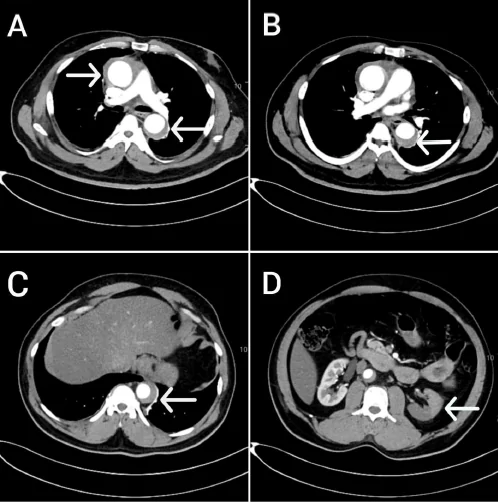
Computed tomography of the chest and abdomen demonstrating Stanford type A aortic dissection. Panel A: periadventitial hematoma surrounding the ascending aorta, extending to the aortic arch and descending thoracic aorta. Panel B: an intramural hematoma in the descending thoracic aorta. Panel C: the descending thoracic aorta with a partially thrombosed false lumen at the level of the thoracic spinal cord. Panel D: a nonopacified left kidney on contrast-enhanced imaging, as the left renal artery arose from the false lumen.
